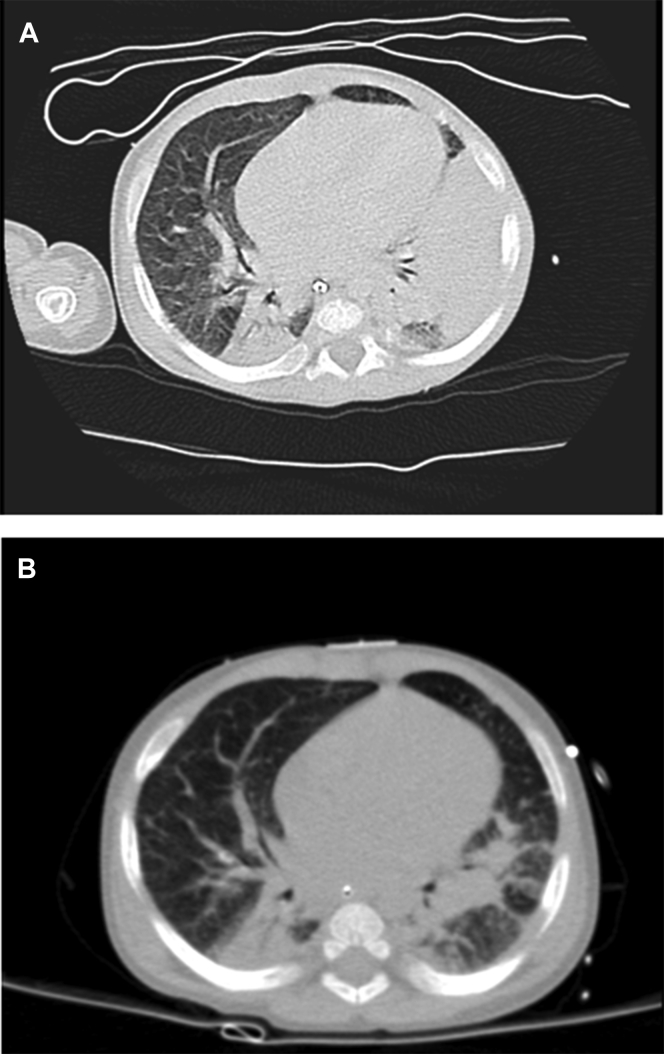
Chest computed tomography scan before (A) and after (B) pioglitazone treatment. Fig 1, *A*, Large left pulmonary consolidation involving the inferior lobe and, partly, the dorsal and lingular segments of the superior lobe, with multiple internal air bronchograms: pneumonia. Similar pulmonary consolidation is present in the right lung and involves the dorsal segments of the inferior lobe. Fig 1, *B*, Remarkable reduction in the areas of consolidation in the left and right lungs; residual pulmonary lesions are visible in the left and right inferior lobes.
